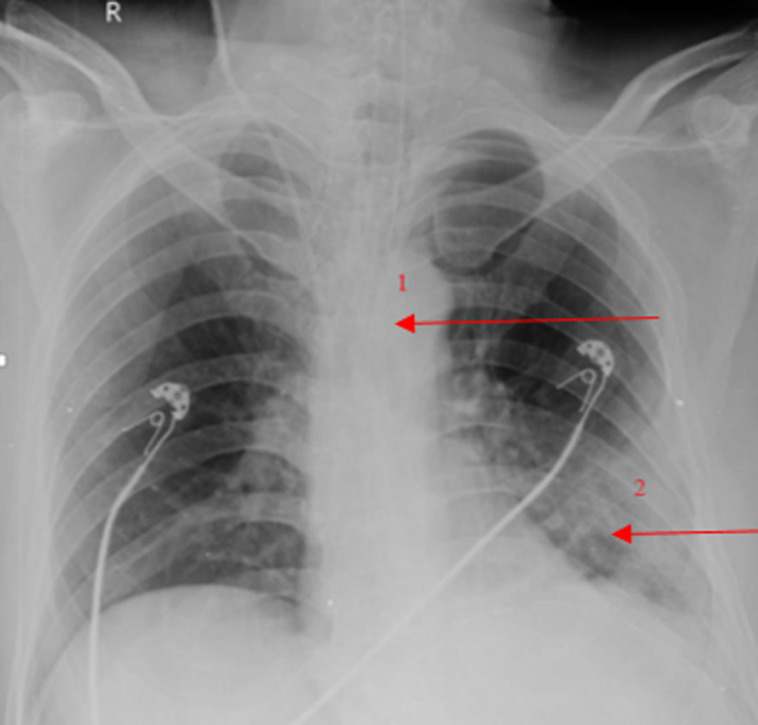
chest x-ray with right mediastinum shift and collapse of left basal lung
